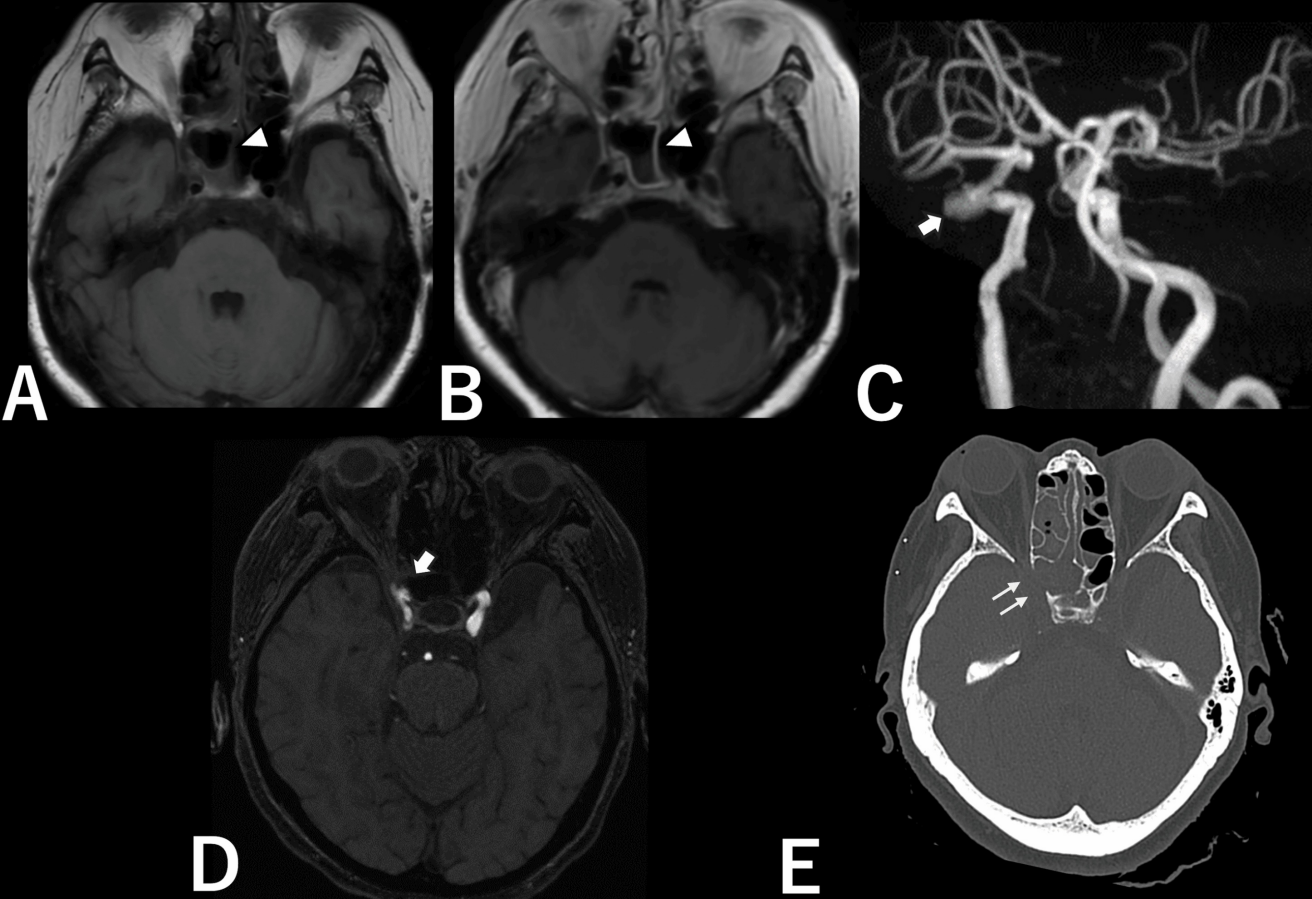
Imaging findings on admission. (A) T1-weighted MRI. (B) Gadolinium-enhanced T1-weighted MRI. White arrowheads in (A) and (B) indicate sinusitis. (C) MRA showing the aneurysm (white arrow). (D) MRA, axial view, showing the aneurysm (white arrow). (E) Head CT showing sphenoid bone loss in the aneurysm area (double white arrow).
